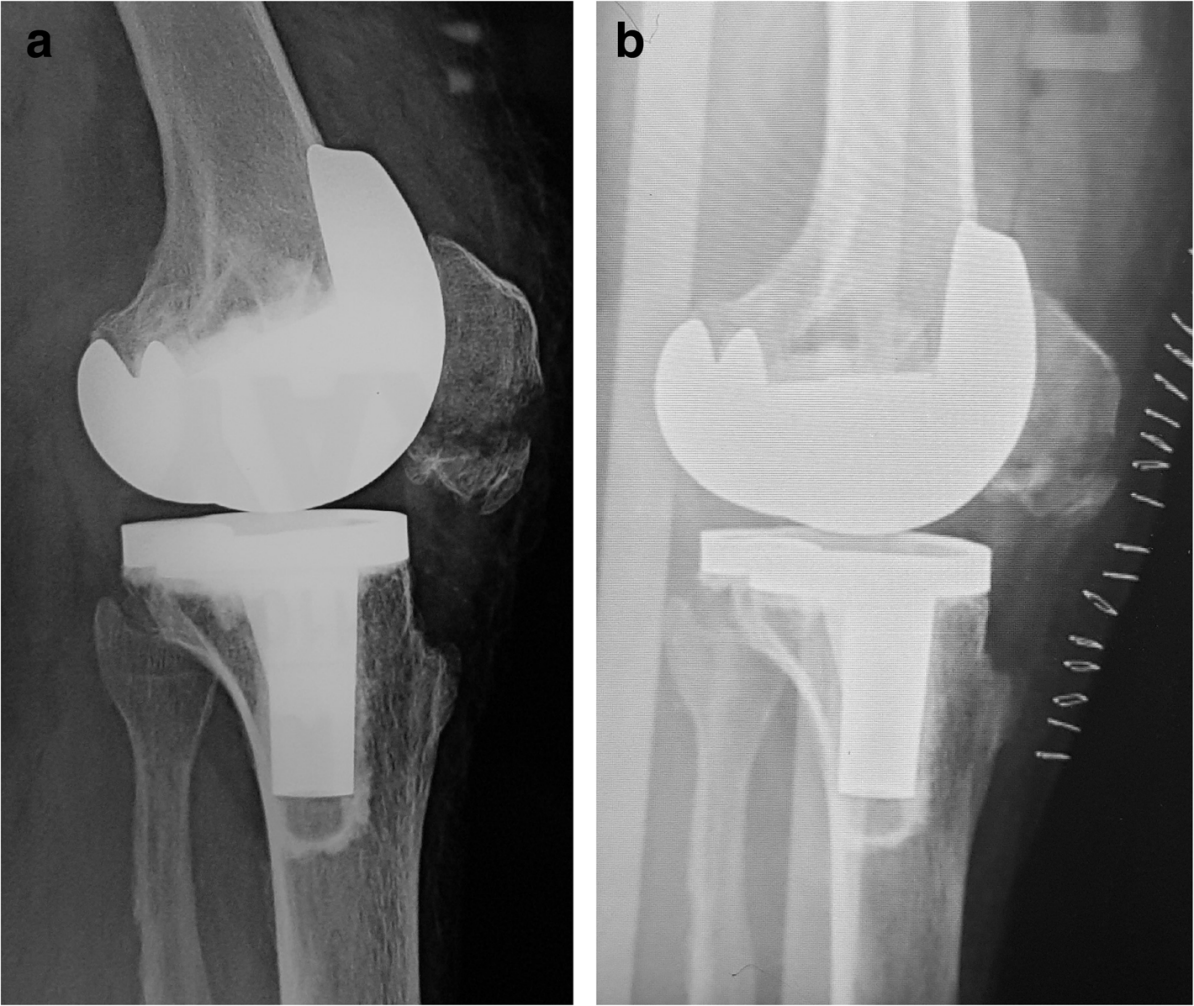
A 65-year-old female patient who had a PPPF in her right knee (case 2 in Table 1). a Preoperative lateral X-ray shows the displaced and comminuted PPPF with extensor mechanism rupture. b Fixation is achieved with Ethibond polyester suture
